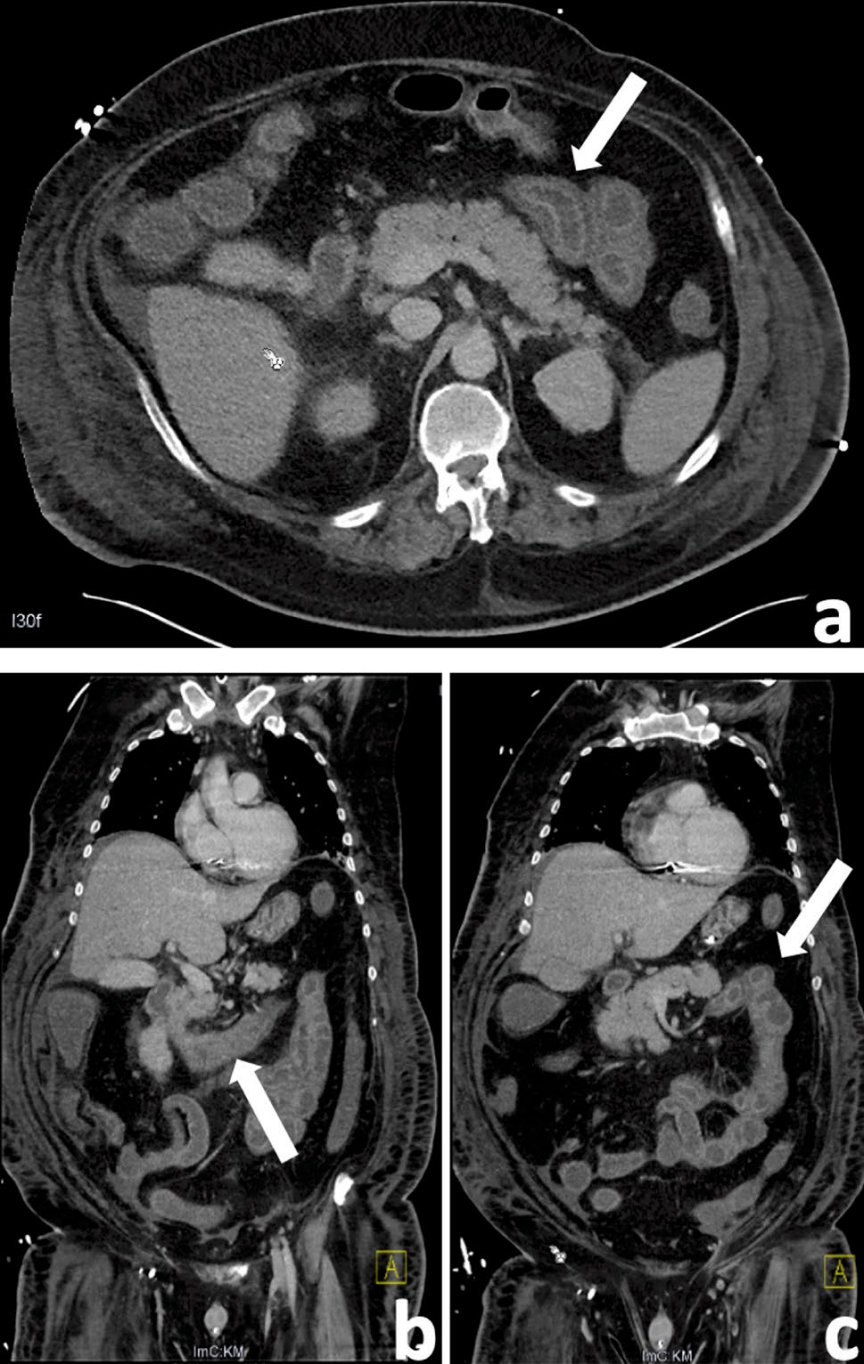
Abdominal contrast-enhanced CT findings of a 48-y male patient with COVID-19 presenting with duodenitis. Axial (A) and coronal (B, C) views show a marked edematous wall thickening in both the proximal and less marked in the ascending duodenum (arrow) and jejunum, while the descending duodenum shows no signs of inflammation, corresponding to the endoscopic finding
